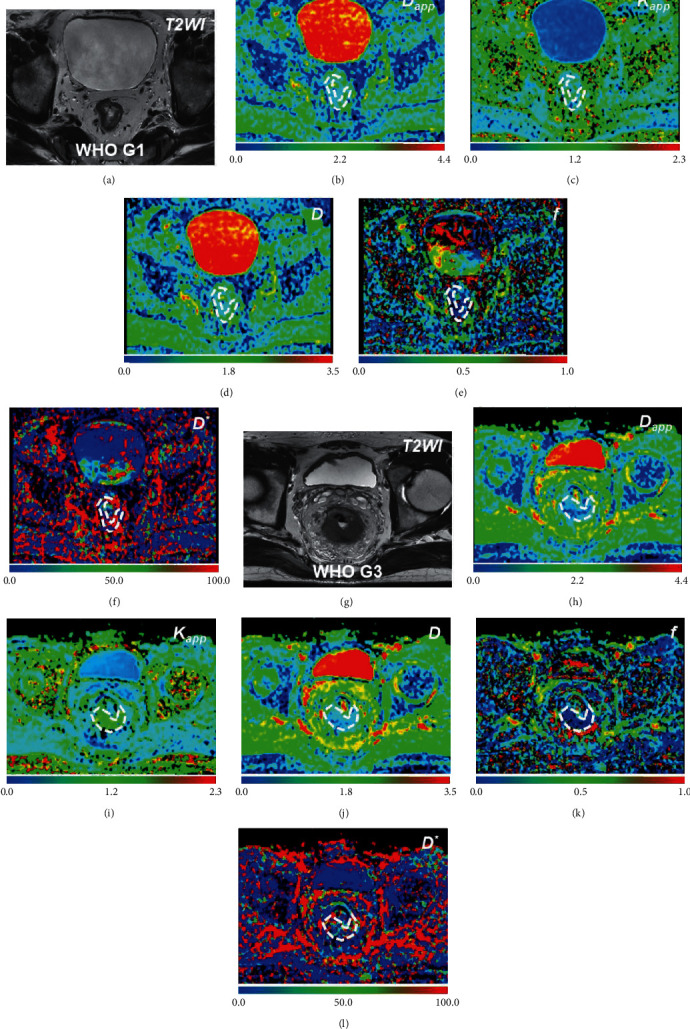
MR images of patients with surgically proven rectal cancer of WHO G1 (a–f) and WHO G3 (g–l). A patient of WHO G1 rectal cancer: (a) T2-weighted image, (b) *D*_app_ image, (c) *K*_app_ image, (d) *D* image, (e) *f* image, and (f) *D*^*∗*^ image. A patient of WHO G3 rectal cancer: (g) T2-weighted image, (h) *D*_app_ image, (i) *K*_app_ image, (j) *D* image, (k) *f* image, and (l) *D*^*∗*^ image. *Note.* The unit of *D D*^*∗*^ and *D*_app_: ×10^−9^ m^2^/s; *K*_app_ and *f* are unitless.
